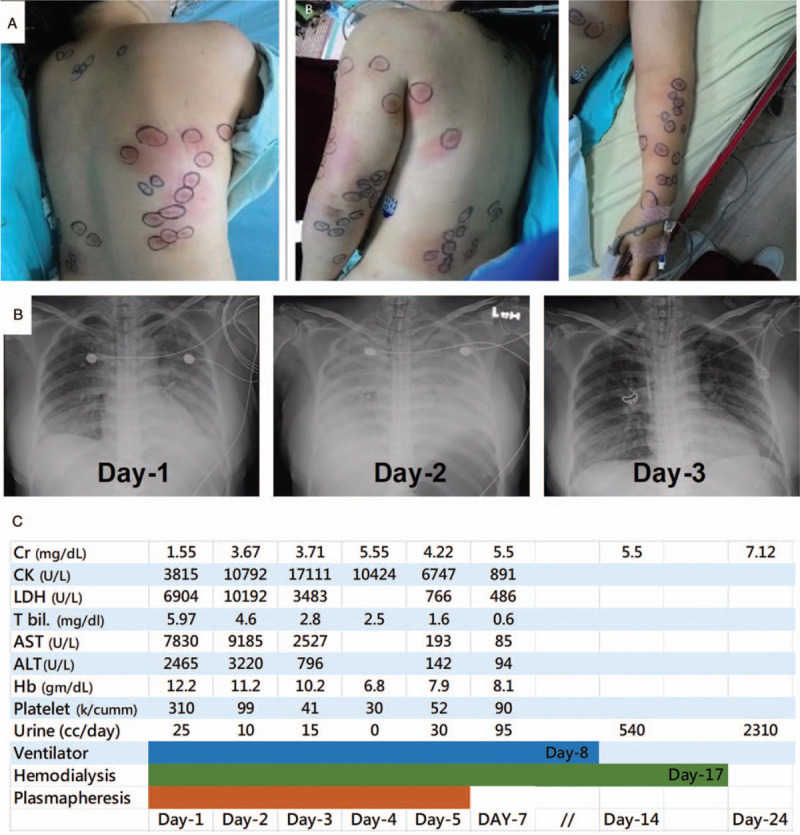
Skin lesions on the trunk (A), serial chest X-ray (B), and serial laboratory findings and management (C) of case 2. ALT =  alanine aminotransferase, AST = aspartate aminotransferase, CK = creatine kinase, Cr = creatinine, Hb = hemoglobin, LDH = lactate dehydrogenase.
